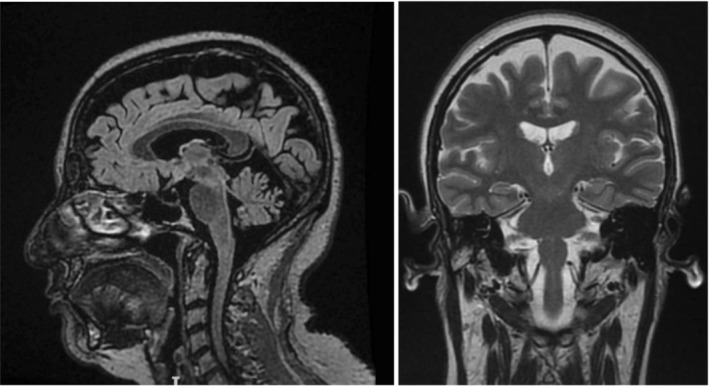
Sagittal 3D‐CUBE FLAIR and coronal T2 FSE brain MRI images showing predominant vermian cerebellar atrophy (sagittal) and enlargement of the pericerebellar and periencephalic subarachnoid spaces (coronal), suggestive of cortical and cerebellar volume loss.
